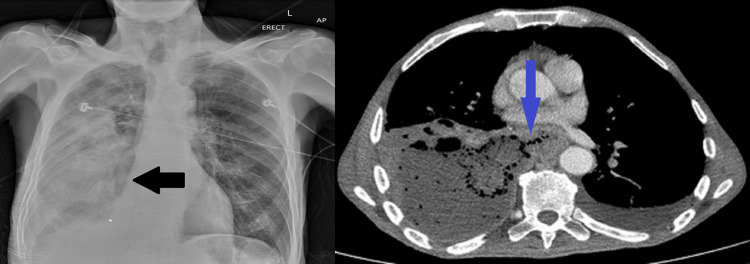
Chest X-ray: right-sided mid-lower zone infiltrates (black arrow); CT thorax: Diffuse oesophageal thickening with right-sided necrotising pneumonia, 14 cm abscess and bronchoesophageal-pleural fistula (BEPF) (blue arrow)
